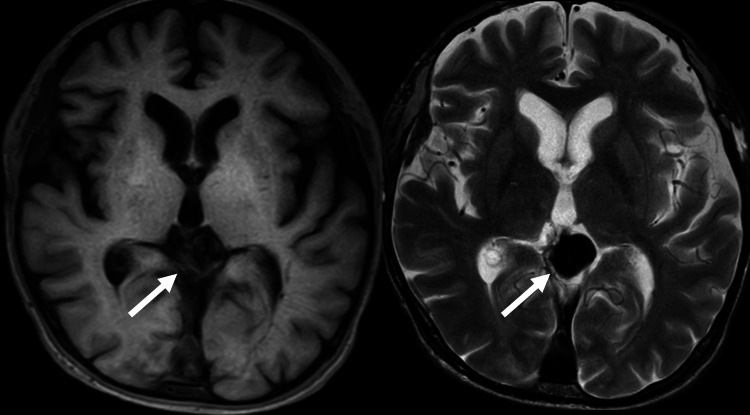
Head magnetic resonance imaging (MRI) on day 8. MRI showed vein of Galen aneurysmal malformation (arrow).
